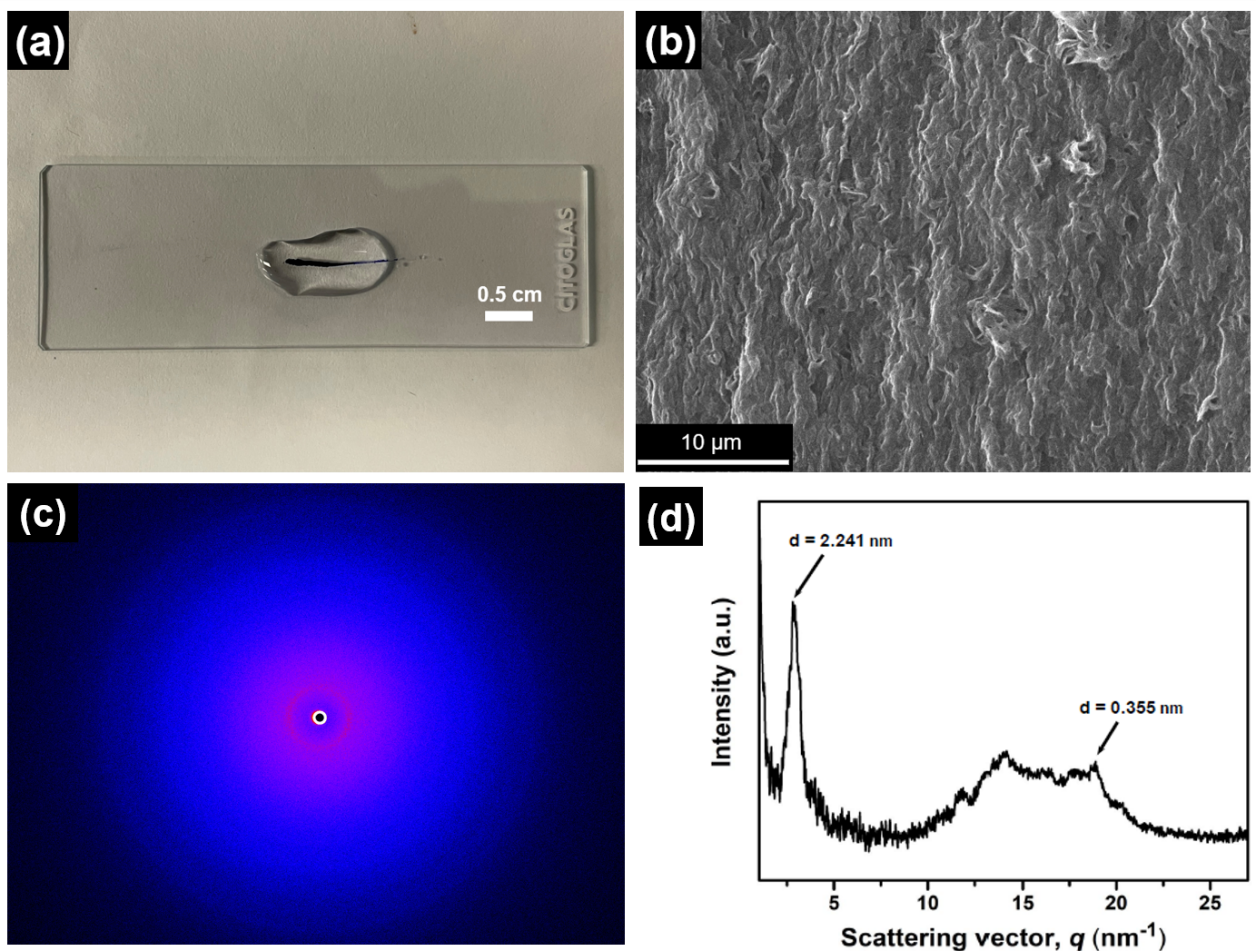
Photograph of a freshly prepared aqueous DA_11_ solution (82.0 mM) ejected into a shallow pool of CaCl_2_ solution (150 mM), (b) SEM image of a macroscopic DA_11_ scaffold prepared from a solution of CaCl_2_ (150 mM), (c) 2D WAXD image of DA_11_ macroscopic soft scaffold with an X-ray exposure time of 20 min, and (d) 1D WAXD pattern of a 2D WAXD image of a DA_11_ macroscopic soft scaffold in (c).
